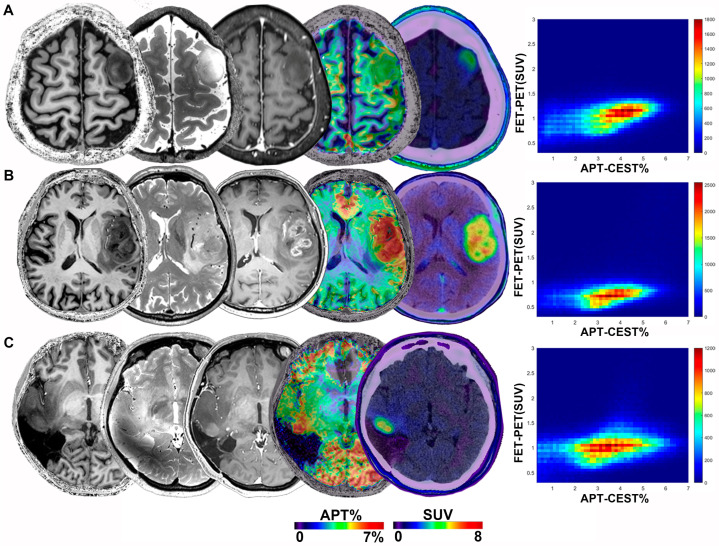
T1-weighted, T2-weighted, contrast-enhanced T1-weighted, APT-weighted MR images, FET-PET images of a 34-year-old male patient with an IDH mutant astrocytoma (WHO Grade II) (A), a 70-year-old male patient with an IDH wildtype glioblastoma (WHO Grade IV) (B) and a 46-year-old female patient with an IDH mutant recurrent astrocytoma (WHO Grade IV) (C). The bivariate histograms between APT values and SUV were present in the right panel, respectively.
